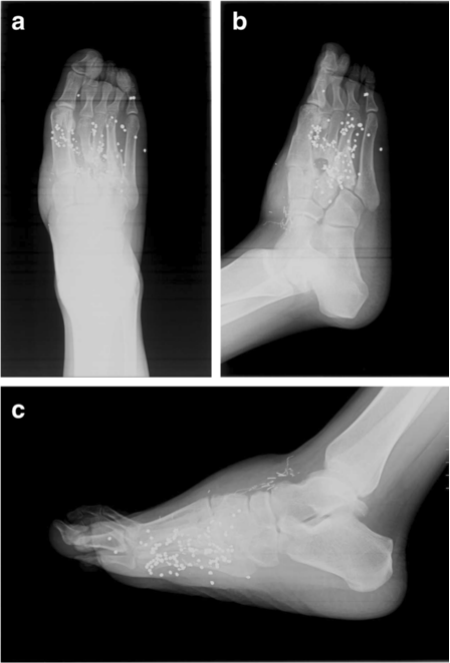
AP (a), oblique (b), and lateral (c) radiographs of the right foot approximately 3 years post-op.
